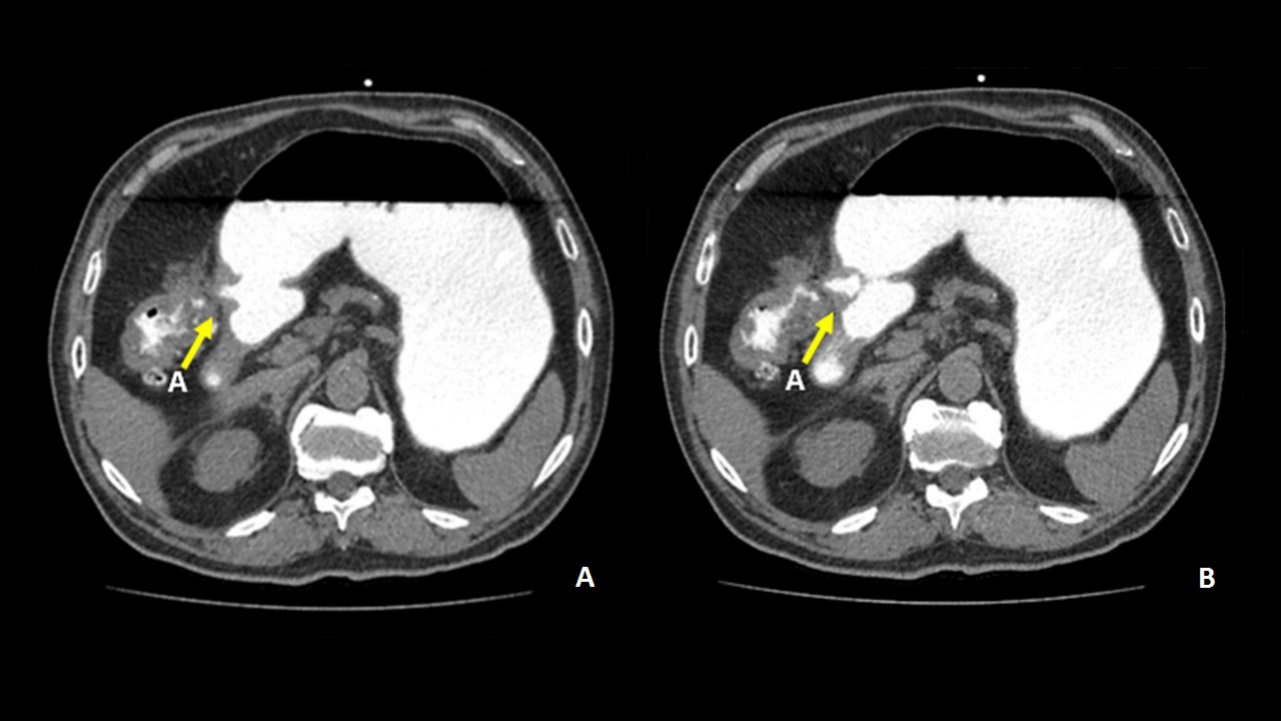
Axial CT scan images demonstrating oral contrast communicating between stomach pylorus and proximal transverse colon (A).
